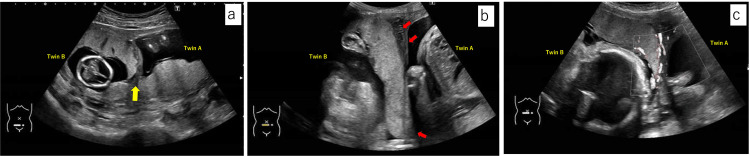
Abdominal ultrasound sonography. (a) Ultrasound image at the gestational age of 14 weeks revealed the placenta of Twin B partially attached to the septum (yellow arrow). (b) Ultrasound image at the gestational age of 28 weeks showed the thinned septum (red arrows). (c) Ultrasound image at the gestational age of 32 weeks showed the flow of the maternal blood floor of Twin B.
